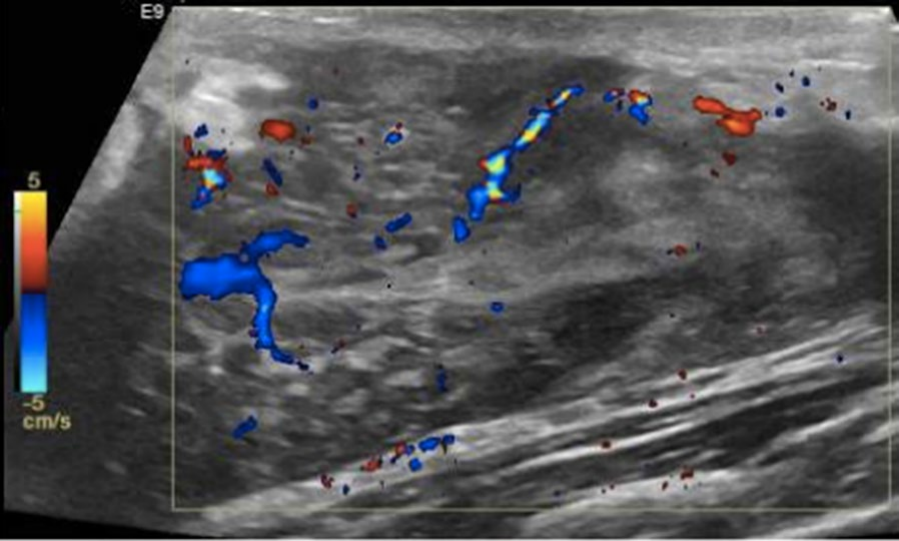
Doppler ultrasound of the patient’s right breast revealed a large, complex area measuring approximately 3.3 cm in thickness with areas of internal vascularity
